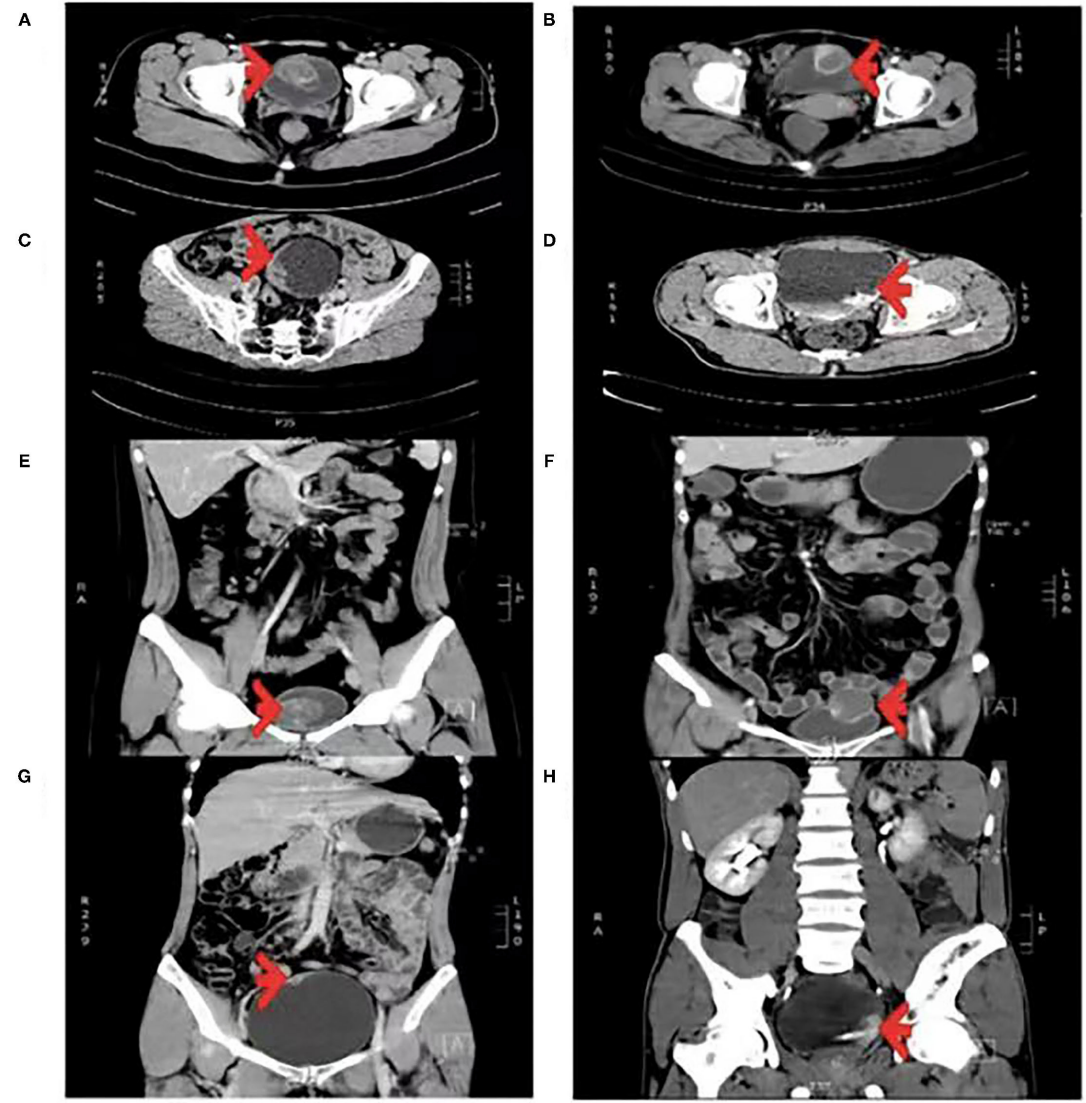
(A–D) Transverse enhanced CT scan. (E–H) Coronal enhanced CT scan. (A,E) Tumor with a size of 43 × 40 mm was located on the anterior wall of the bladder, papillary protrusion into the cavity with ring enhancement was present, and the CT value was 53 HU. (B,F) Tumor with a size of 36 × 33 mm was located on the anterior-superior wall of the bladder, exophytic growth with ring enhancement was present, and the CT value was 54 HU. (C,G) Tumor with a size of 48 × 12 mm was located on the right lateral wall of the bladder, papillary protrusion into the cavity was present, and the CT value was 59 HU. (D,H) Tumor with a size of 27 × 21 mm was located on the left lateral wall of the bladder, papillary protrusion into the cavity and suspected invasion to the left ureter were present, and the CT value was 59 HU.
